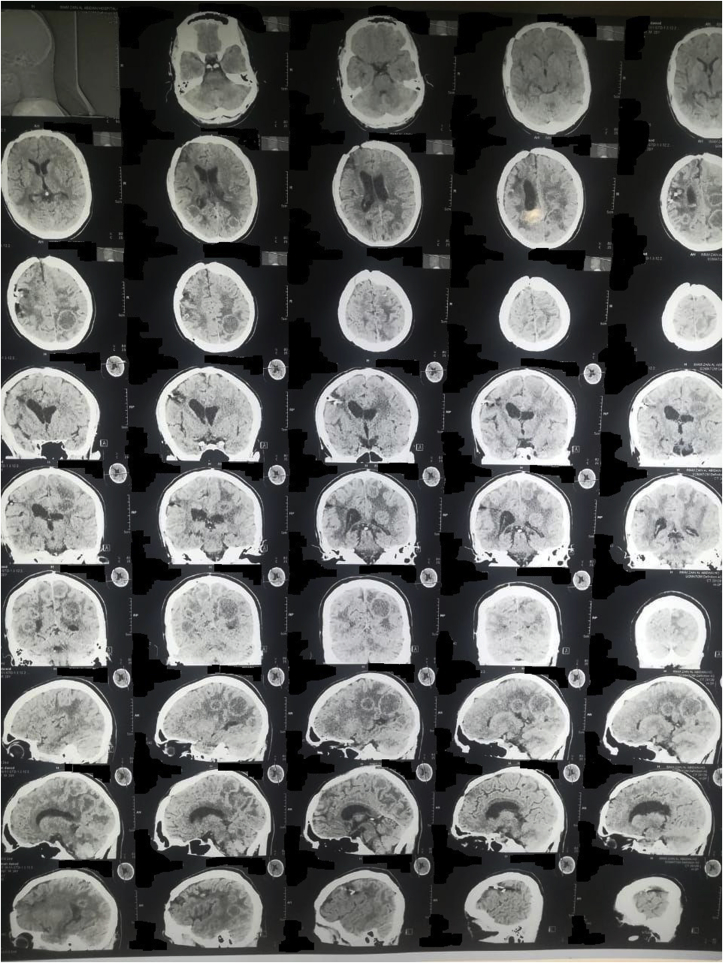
CT Scan brain 10 months postoperative brain surgery.
